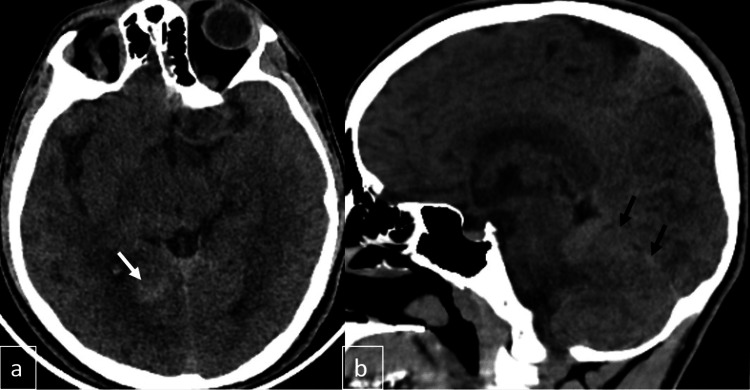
Axial CT image (a) shows a poorly defined hyperdense area in the right posterior parahippocampal region, while sagittal CT image (b) demonstrates mild cerebellar parenchymal hyperdensity with folial effacement suggestive of edema. CT: computed tomography
